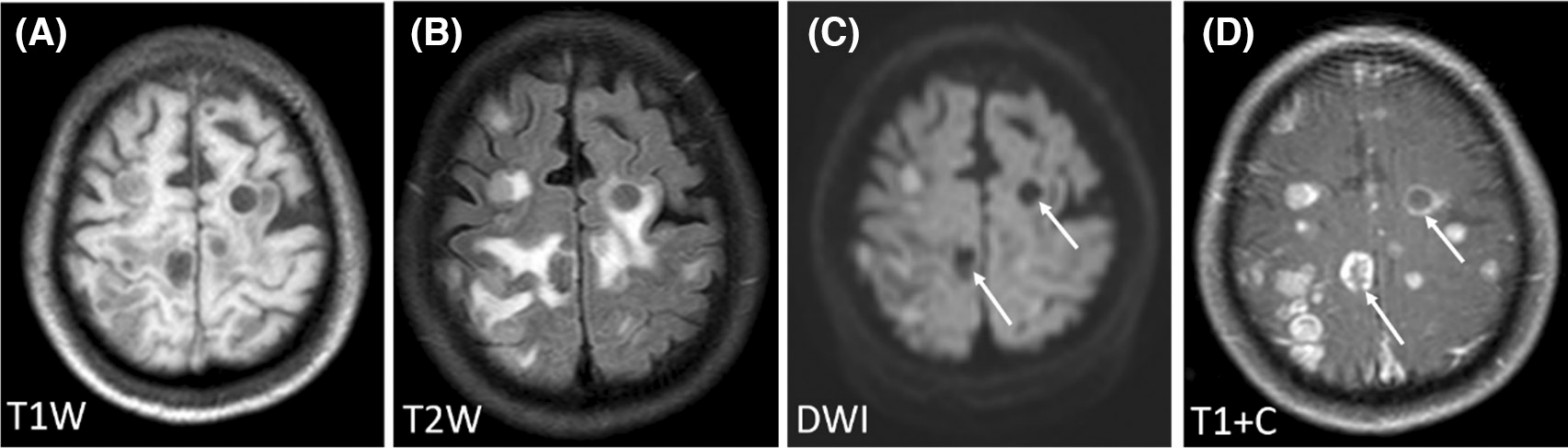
Pattern VI of brain metastases in SCLC. Pattern VI: A 77‐year‐old woman without history of smoking was diagnosed with BMs of SCLC. The patient had numerous lesions located in the supratentorial and infratentorial areas. The lesions (white arrows) were located in the frontal lobe and centrum semiovale, with hypointensity in T1WI (A), hypointensity in T2WI (B), hypointensity in DWI (C) and homogeneous and ring enhancement (D). BMs, brain metastases; DWI, diffusion‐weighted imaging; SCLC, small cell lung cancer.
